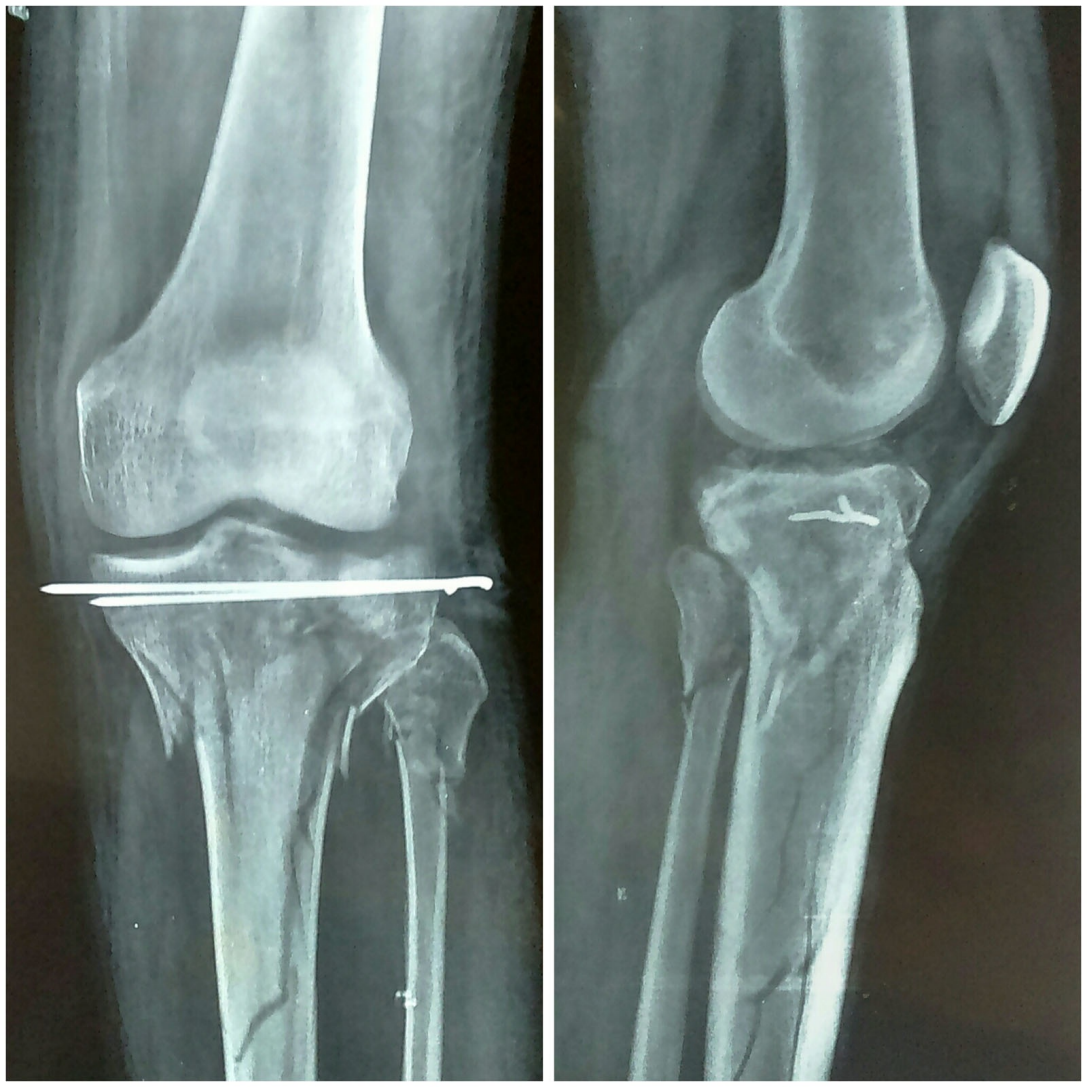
Immediate postoperative X-ray views: anteroposterior and lateral.
